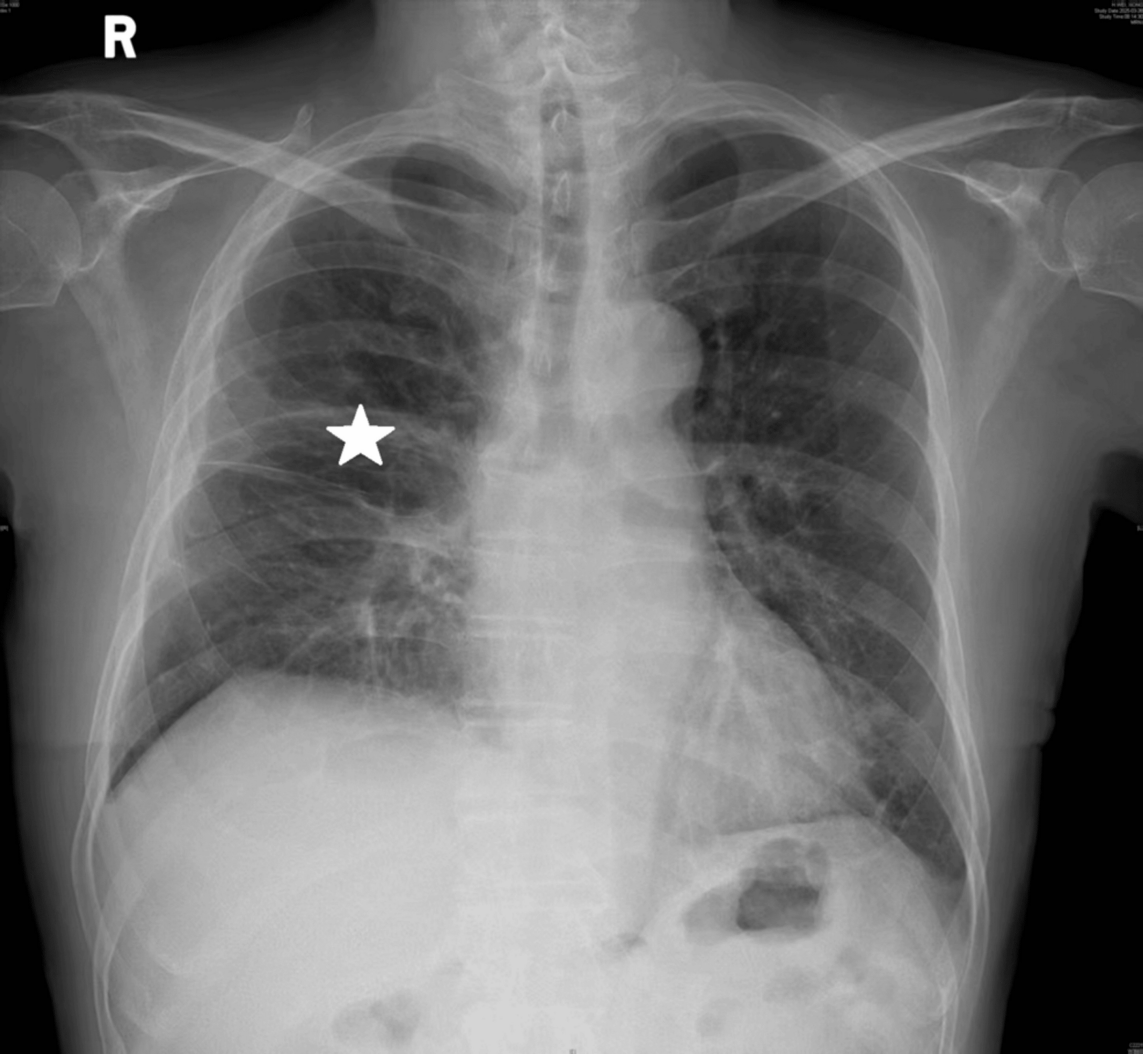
Pre-discharge Chest X-ray A portable chest radiograph reveals near-complete resolution of the right pyopneumothorax and satisfactory re-expansion of the right lung (asterisk).
